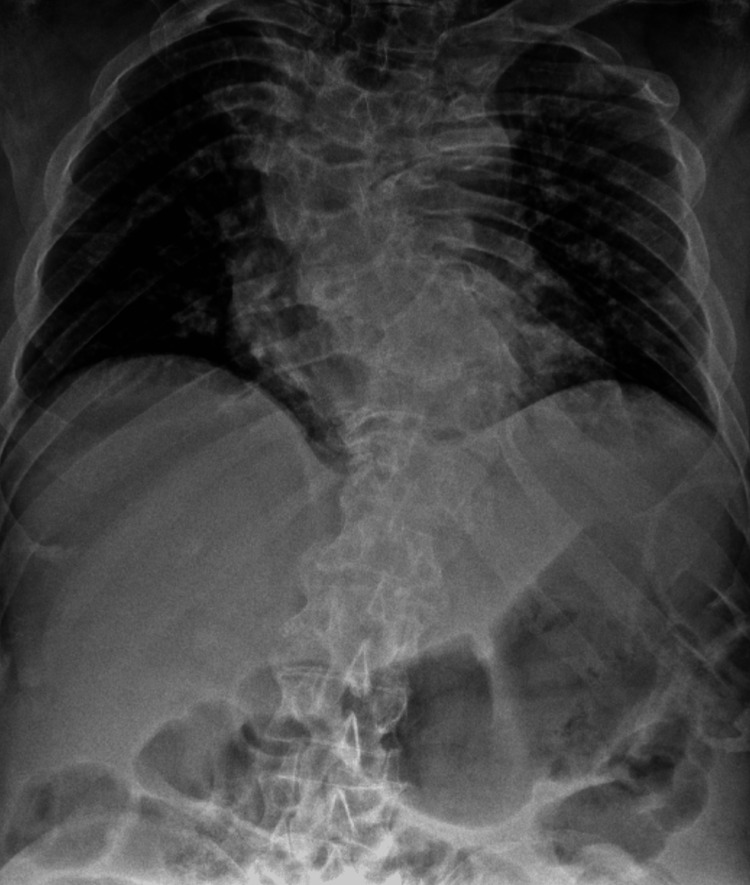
Chest X-ray demonstrating thoracolumbar scoliosis.
